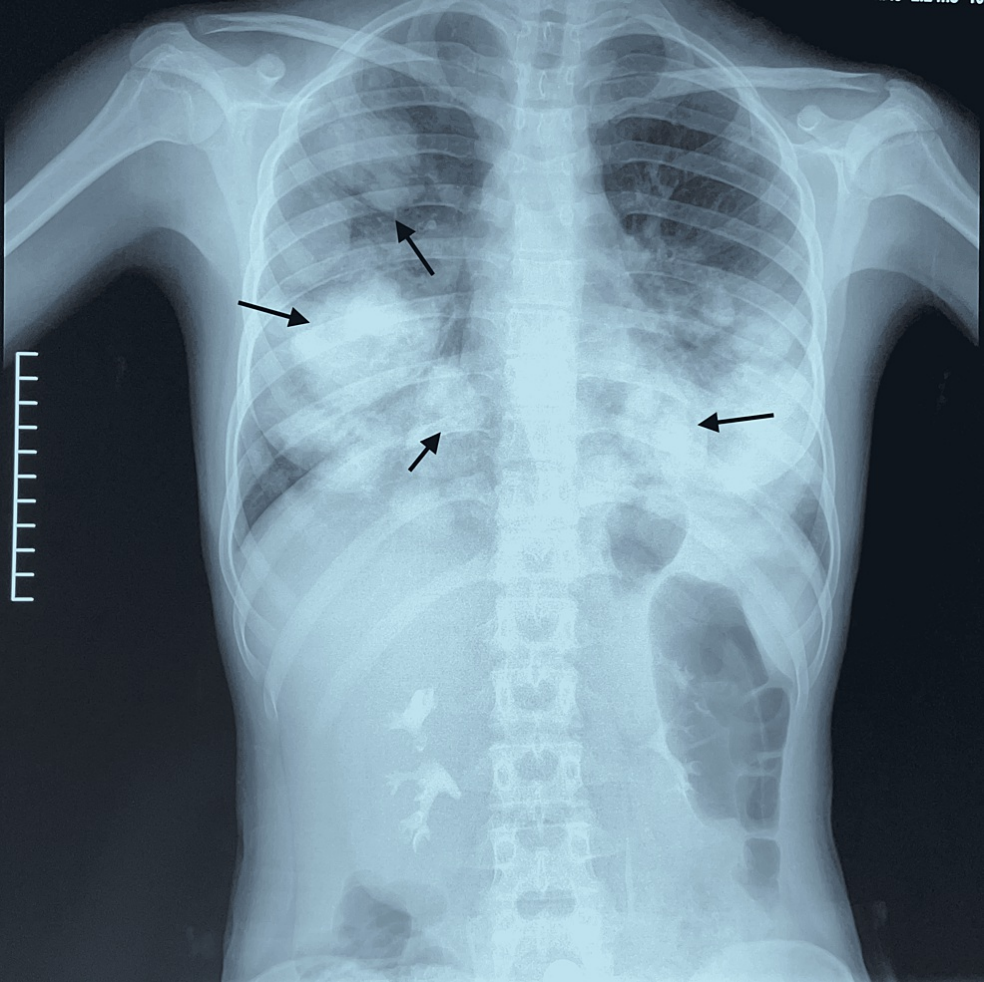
Chest x-rays of the patient, a 14-year-old girl, showed bilateral rounded nodules with cannonball appearance (arrows), highly evocative of pulmonary metastases.
